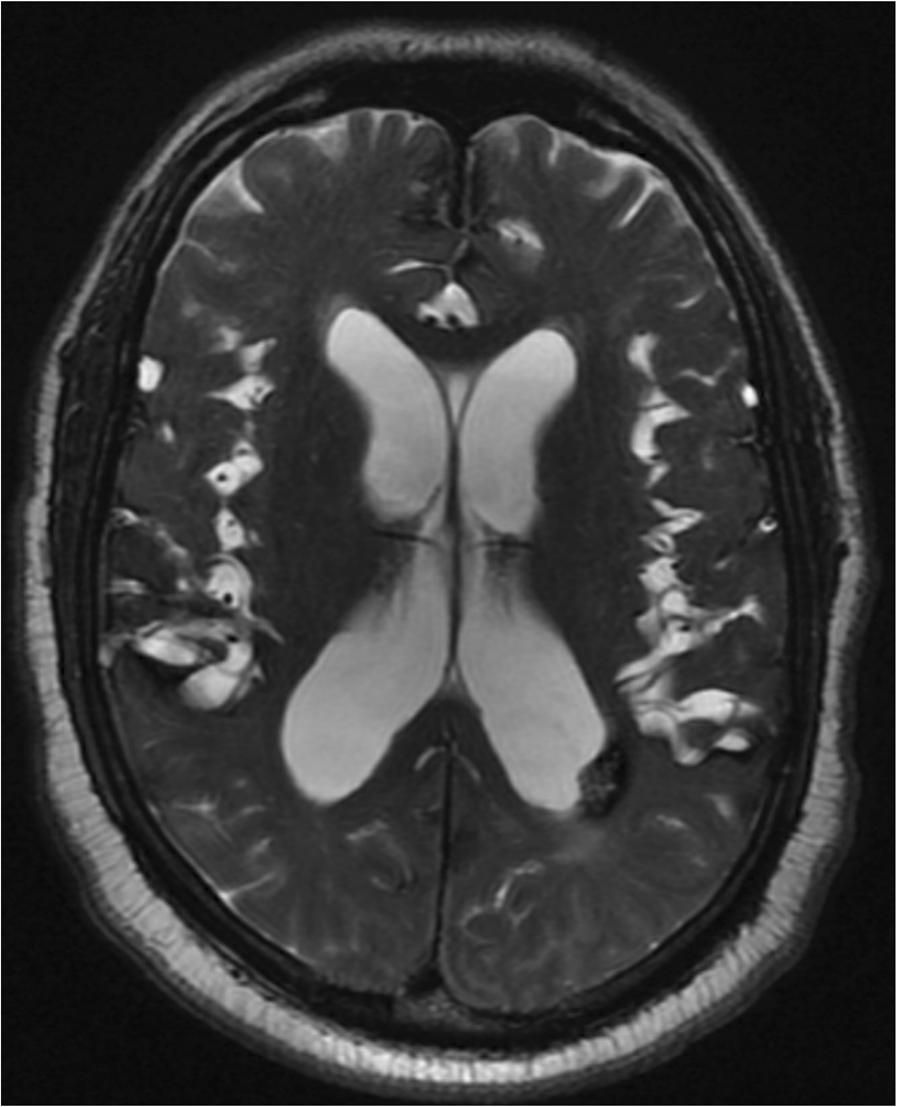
T2 weighted MRI brain without contrast on admission, showing peri-atrial cavernous malformation and hydrocephalus
